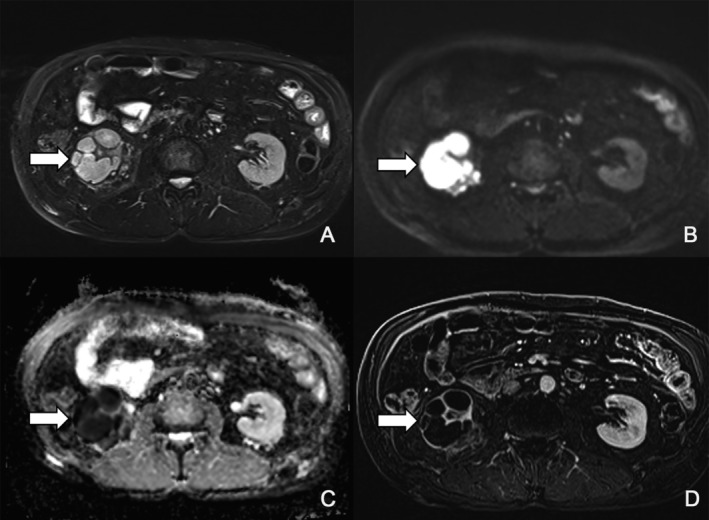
Renal Magnetic resonance imaging (MRI) findings. (A) An axial T2‐weighted fat‐suppressed image shows a multiseptated mass in the right kidney with heterogeneous high signal intensity. (B, C) The mass exhibits diffusion restriction on an axial diffusion‐weighted image (DWI) (*b*‐value = 1000 s/mm^2^) and the corresponding apparent diffusion coefficient (ADC) map. (D) The mass demonstrates thick septal and wall enhancement on an axial postcontrast subtraction image acquired at 60 s.
